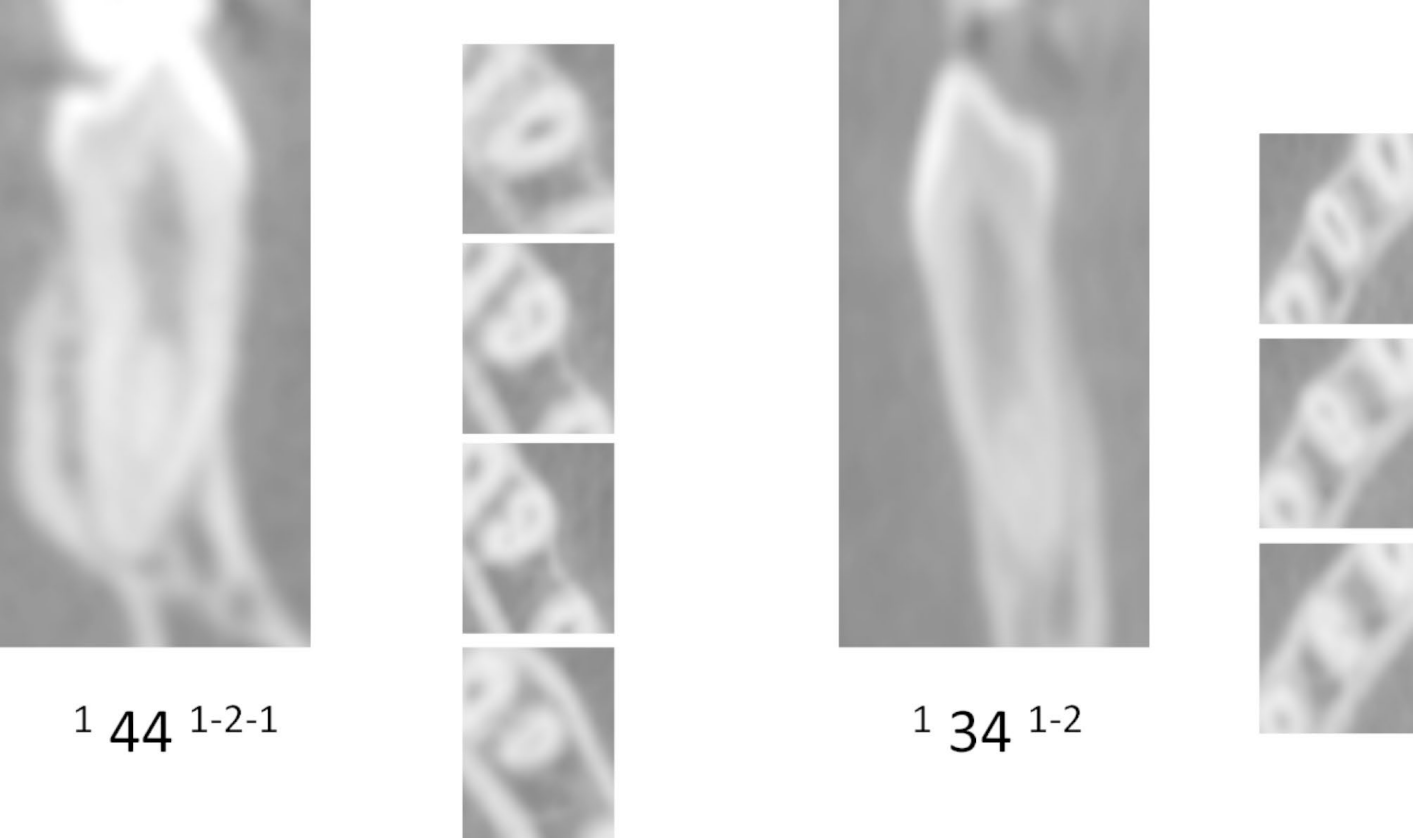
CBCT View (Sagittal and axial) of mandibular first premolars showing the canal variations
